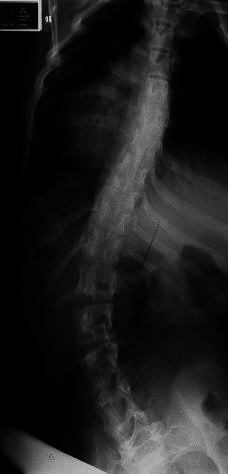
Pre and postoperative radiographs of a patient with Duchenne myopathy treated by posterior spinal arthrodesis using the Unit Rod. In this case, the pelvic obliquity was of 30° before the surgery and was reduced to 0° postoperatively
